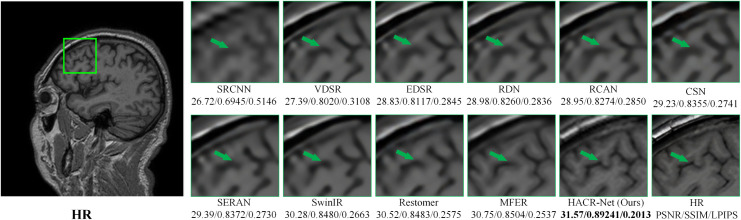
Visual comparison of different SR methods on the T1-weighted IXI dataset at × 4 magnification. The green box indicates the zoomed-in region, where local textures and fine anatomical structures can be clearly assessed.
